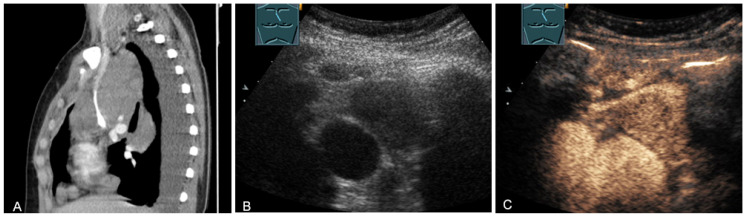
A young patient with tumor formation in the anterior mediastinum on CT (provided by Prof. Dr. Andreas H. Mahnken, Marburg, Germany) (A), B-mode US (B), and CEUS (C), confirmed by biopsy as Hodgkin’s disease.
